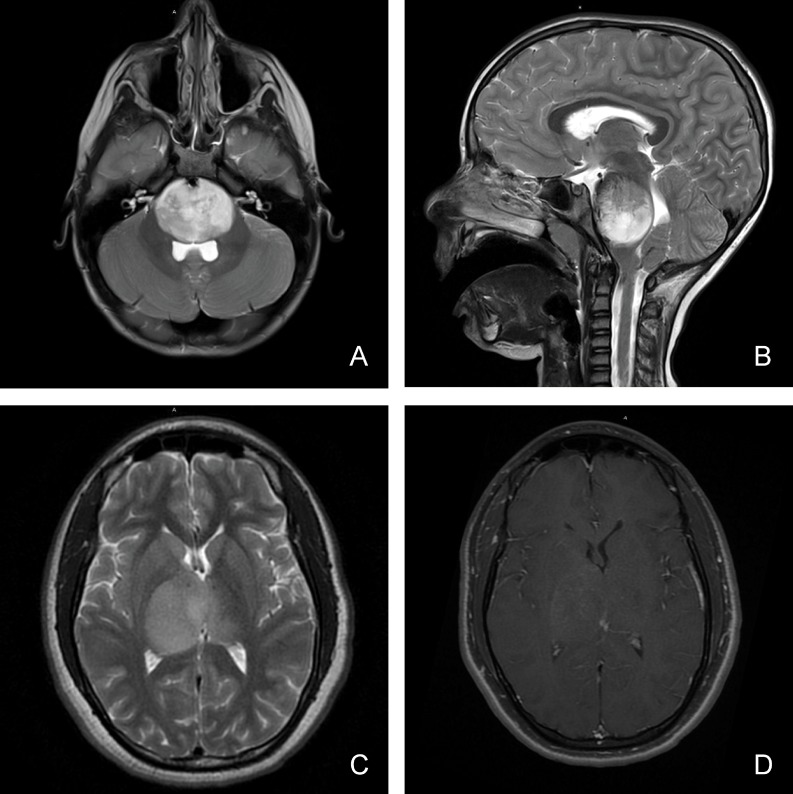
Magnetic resonance imaging characteristics (MRI) of pediatric diffuse midline glioma Pediatric diffuse midline glioma arise in the brainstem (A, B) or thalamus (C, D). T2 weighted MR imaging (A, B, C) demonstrates a homogenous, expansile, infiltrative lesion with extension within white matter tracts. Minimal to heterogenous enhancement may be observed on T1 post-gadolinium sequences (D). Local mass effect and perilesional edema may also be seen.
